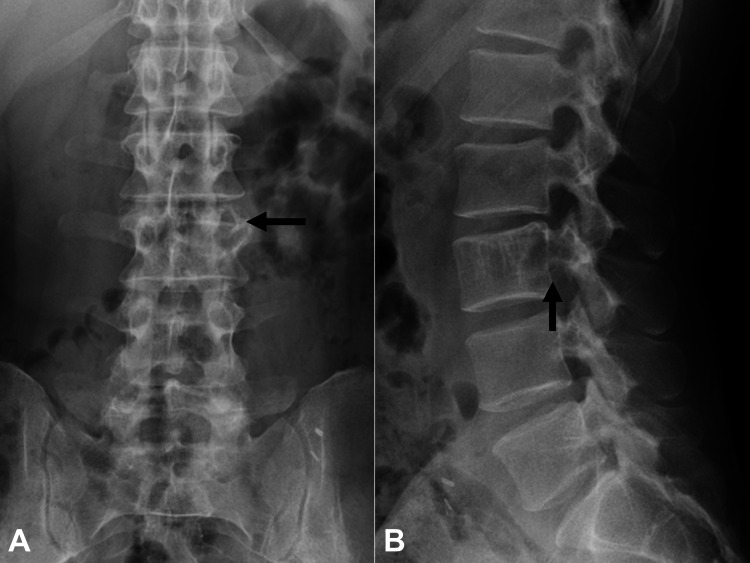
Plain lumbar radiographs of the lumbar spine. Osteolytic lesion involving the left L3 posterior portion of the vertebra with a pedicle sign (arrows). (A) Anterior-posterior view. (B) Medial-lateral view.
